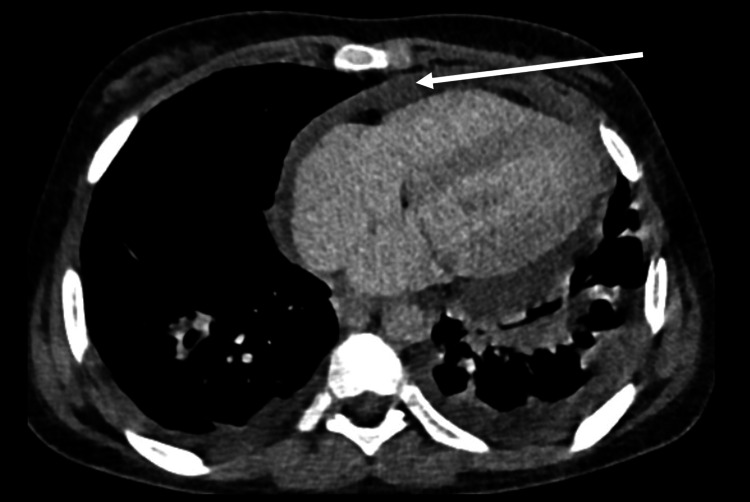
Contrast-enhanced computed tomography (CECT) chest axial cuts showing pericardial effusion (white arrow)
